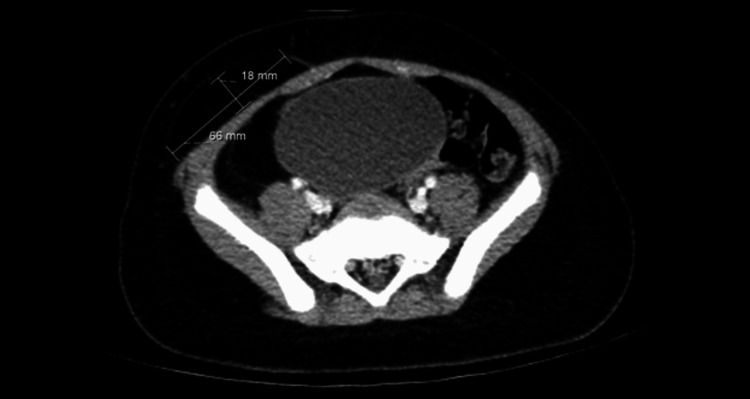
Initial CT abdomen and pelvis – image 2 Focal area of fat in the right lower quadrant anterior to the abdominal wall measuring 6.6 x 1.8 cm consistent with lipoma CT: computed tomography
